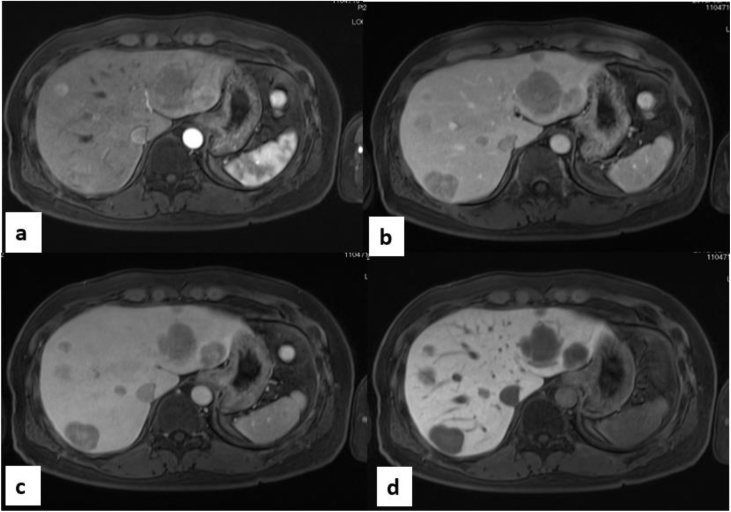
Gd-EOB-DTPA-MRI imaging. Gd-EOB-DTPA-MRI demonstrated multiple metastases (11 metastases) in both liver lobes (arrows). (a) arterial phase (b) portal phase (c) equilibrium phase (d) hepatobiliary phase
